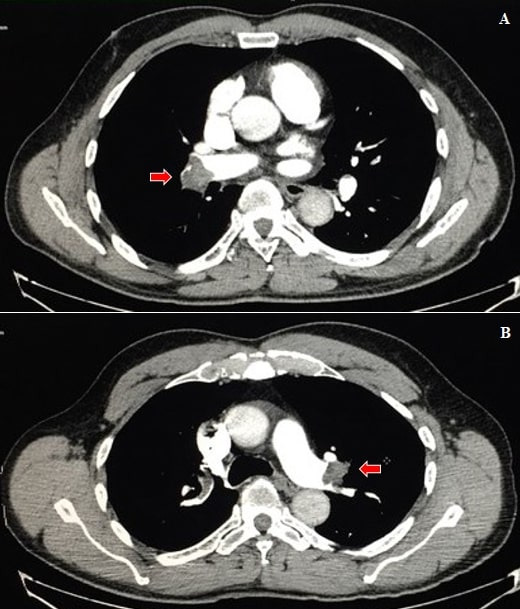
Computed tomography angiography of the chest, axial plane, showing failure to completely fill right (A) and left (B) pulmonary arteries because of obstruction by thrombus (arrows).
